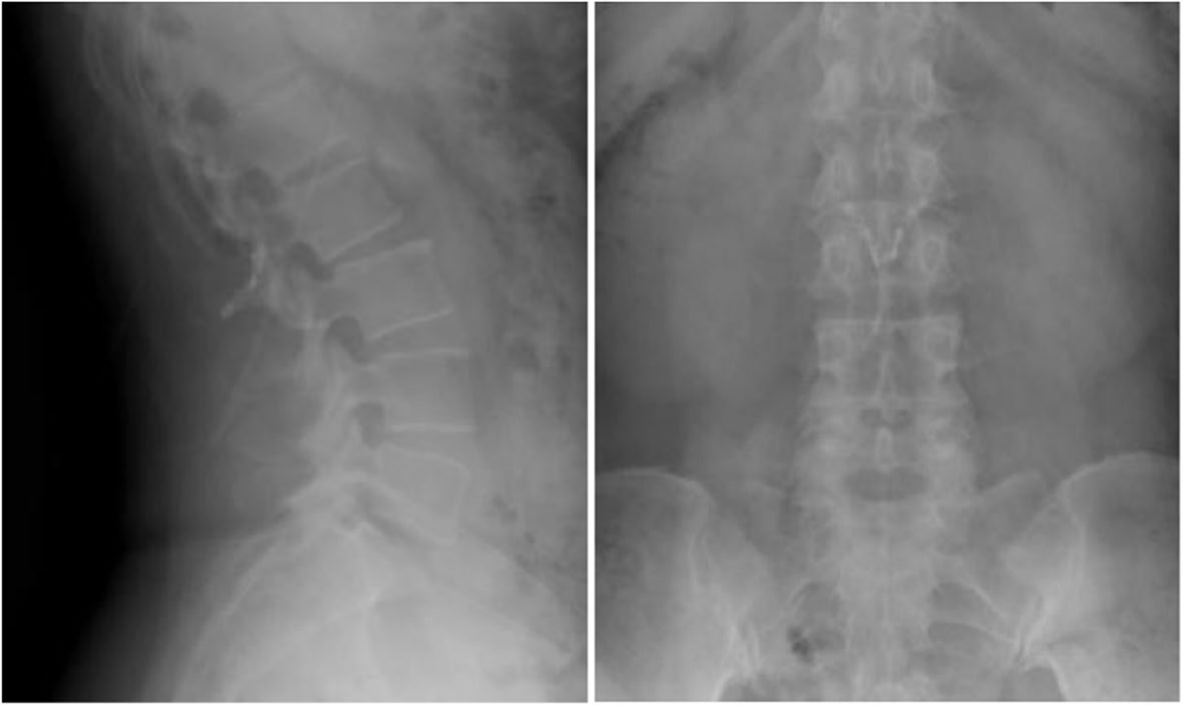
One month after operation, the positive X-ray plain film of lumbar showed that the replanted lamina began to heal
